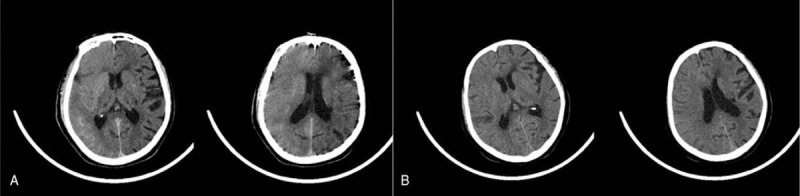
A. CT showed obvious swelling in the right hemisphere with high-density images, which was like subarachnoid hemorrhage. B. CT on the second day showed that the edema in the right hemisphere was significantly improved and the high-density image disappeared. CT = computed tomography.
